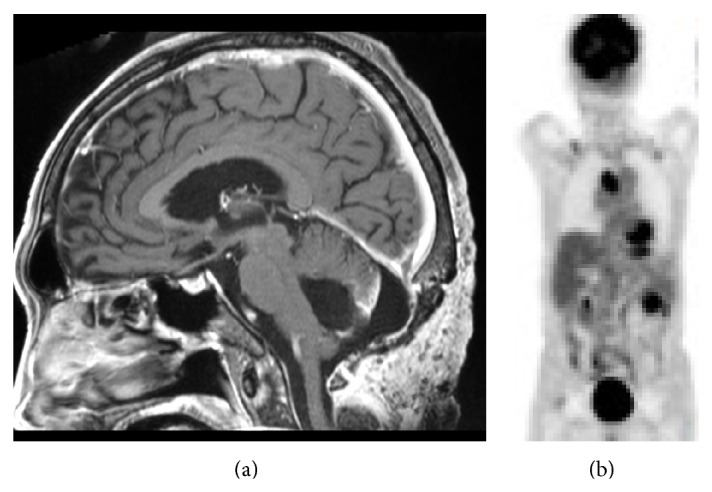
Postoperative MRI shows medulla decompressed without the residual tumor in the vermis (a). Positron emission tomography (PET) reveals abnormal accumulations in the cardiac apex, subclavial region, and peritoneal cavity (b).
